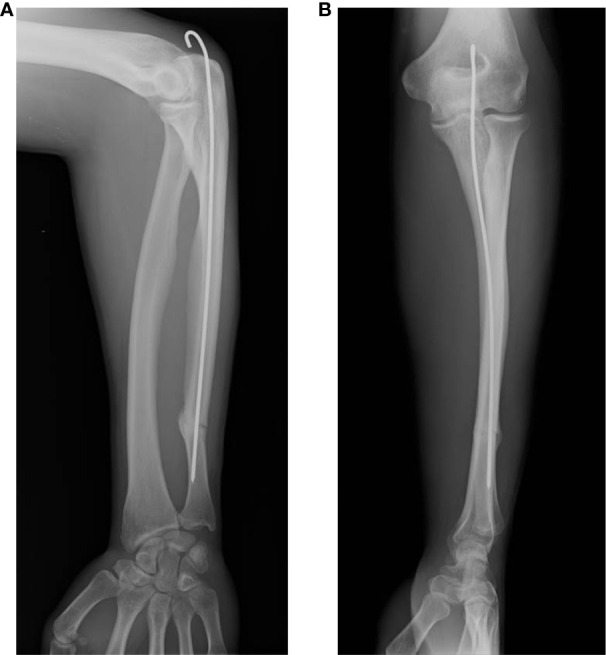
Radiographic consolidation at the fracture site after ESWT treatment. The presence of callus is visible on anterior–posterior (A) and lateral (B) X-rays of the left ulna.
